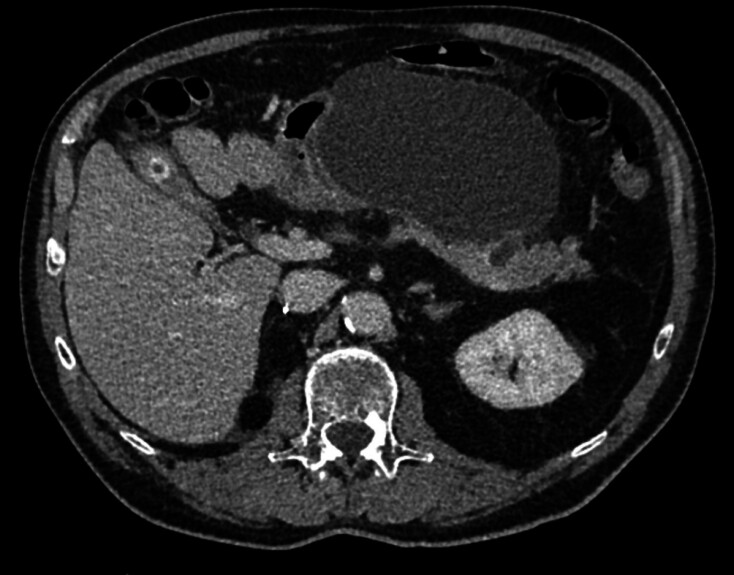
Abdominal CT scan of a pancreatic fistula with retro gastric pseudocyst, 5 weeks after endoscopic ultrasound-guided radiofrequency ablation in Patient 3.
